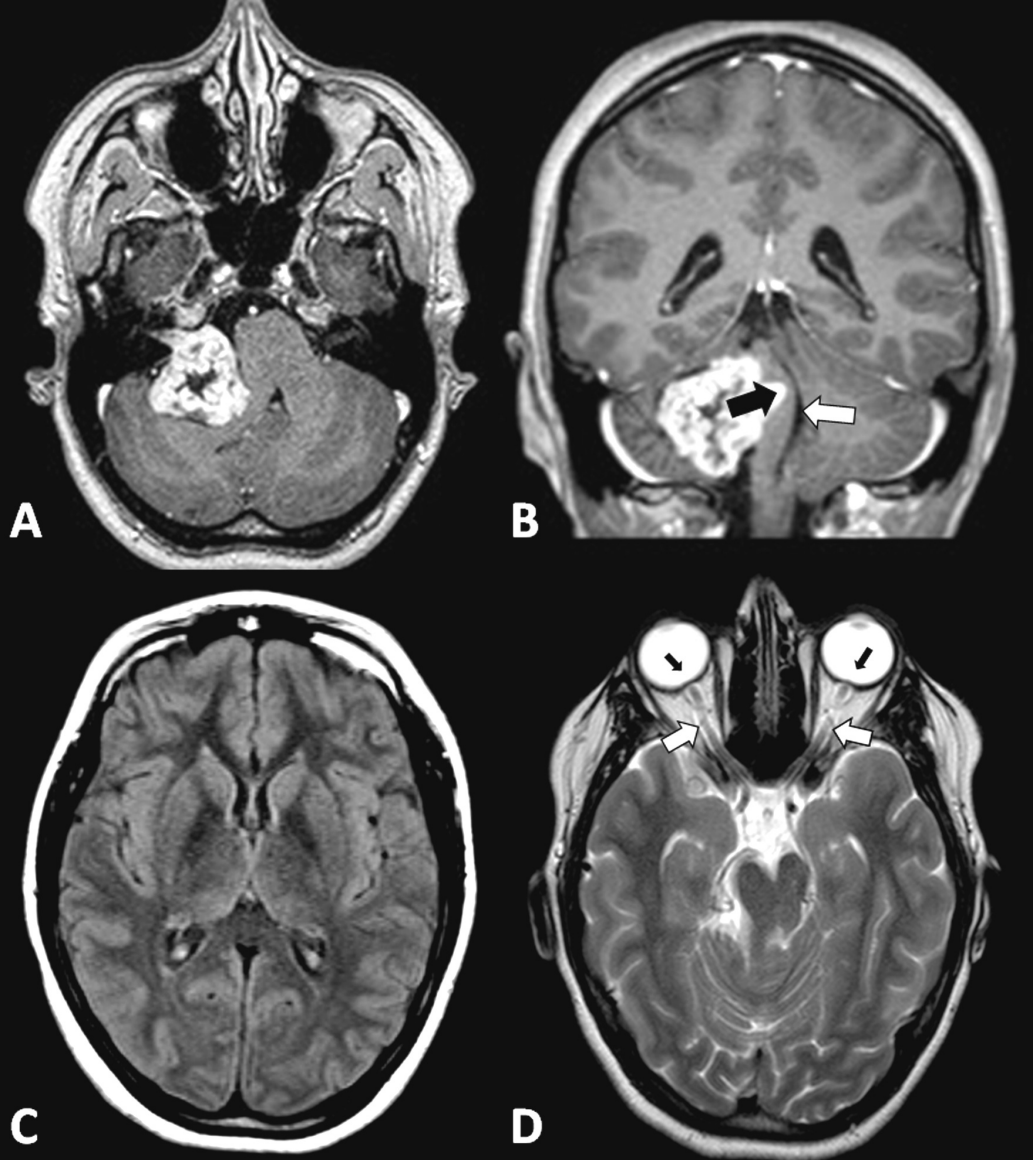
Preoperative magnetic resonance imaging (MRI) (A) Axial T1-weighted gadolinium-enhanced MRI showing a sharply marginated non-homogeneously enhancing right cerebellopontine angle (CPA) mass extending into the right internal auditory canal in a 20-year-old woman. (B) Coronal T1-weighted gadolinium-enhanced MRI showing compression on the brainstem from tumor mass effect (black arrow), with a patent aqueduct of Sylvius (white arrow). (C) Axial T2-weighted fluid-attenuated inversion recovery (FLAIR) MRI showing normal ventricle size without signs of transependymal edema. (D) Axial T2-weighted MRI. The hyperintense signal surrounding the optic nerves (white arrows) is due to increased CSF pressure leading to dilatation of the paraneural subarachnoid space. Note flattening of the posterior aspect of the globes (black arrows).
